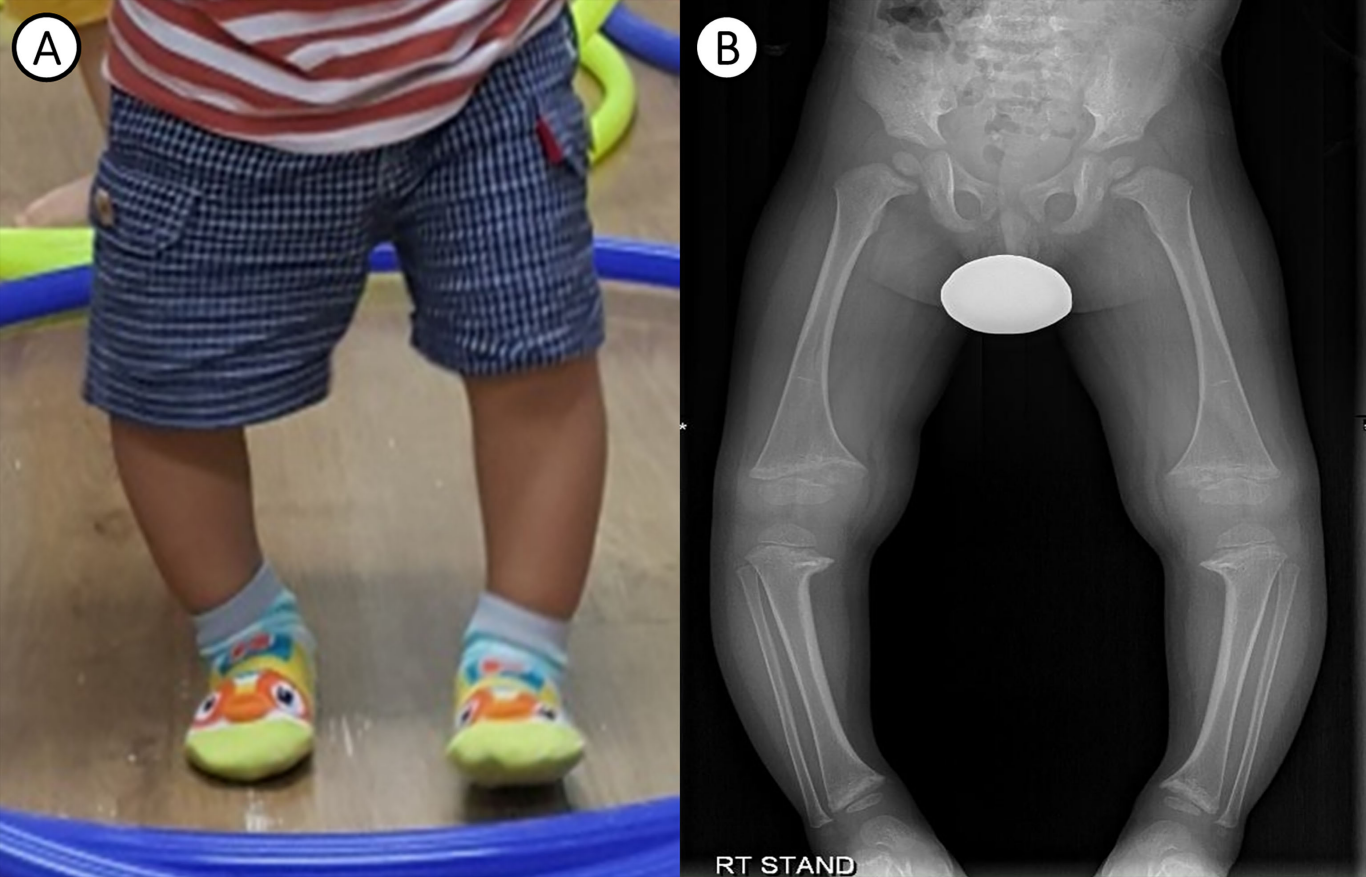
Clinical photograph and radiological findings of the patient. (A) Photograph of the patient’s bowed legs at 24 months of age. (B) Plain radiograph of the lower extremities showing bilateral metaphyseal flaring of the distal femur and tibia, associated with varus deformity, at 30 months of age.
